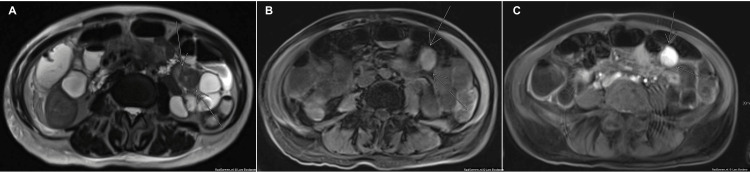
MRI scanning results A) MRI T2 haste axial image showing T2 hypointense mesenteric mass. B) MRI T1 fat-saturated pre-contrast axial image showing T1 isointense mesenteric mass. C) MRI T1 fat-saturated post-contrast axial image showing enhancing mesenteric mass.
